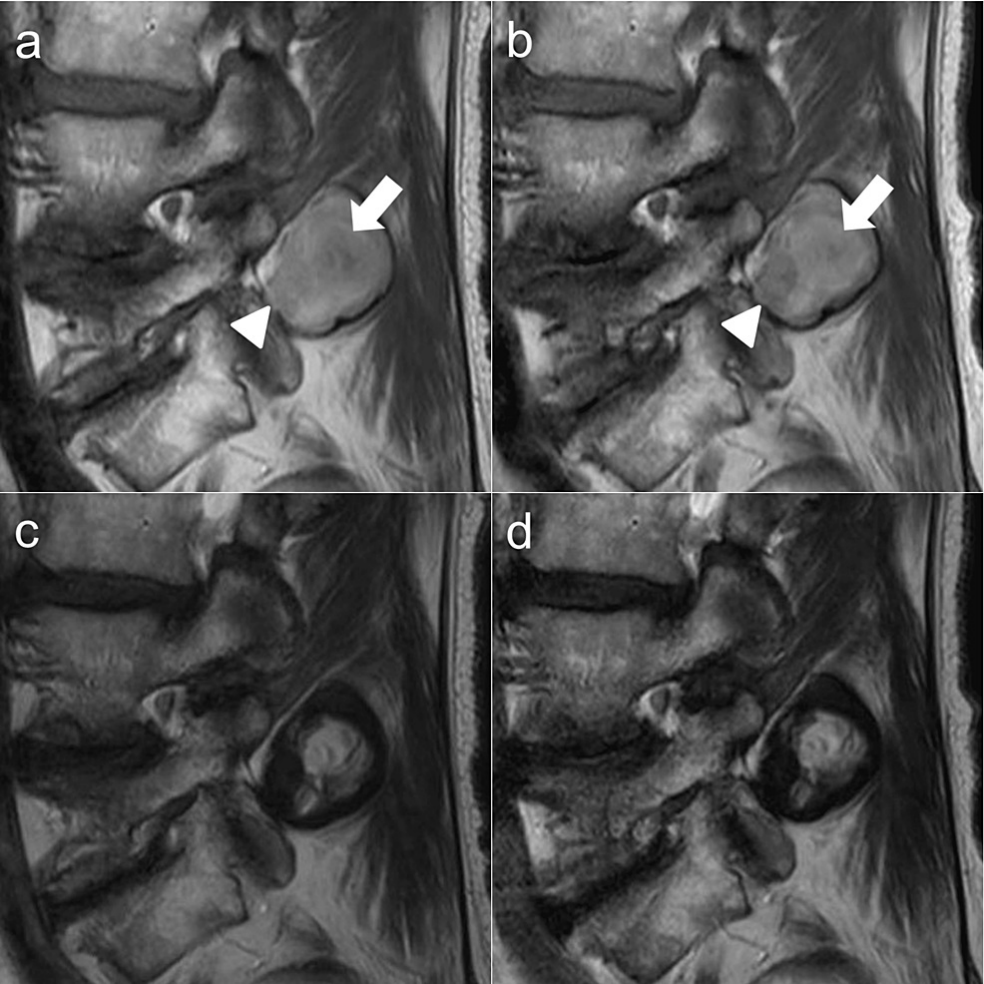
(a, b) Parasagittal MRI of extraspinal space-occupying lesions in the multifidus at the L4–L5 facet level on the 13th (left column) and 21st days of hospitalization (right column); (c, d) Sagittal T2-weighted MRI shows almost no signal changes. Signal changes of the dorsorostral region are indicated by arrows and those of the ventrocaudal area are indicated by arrowheads
